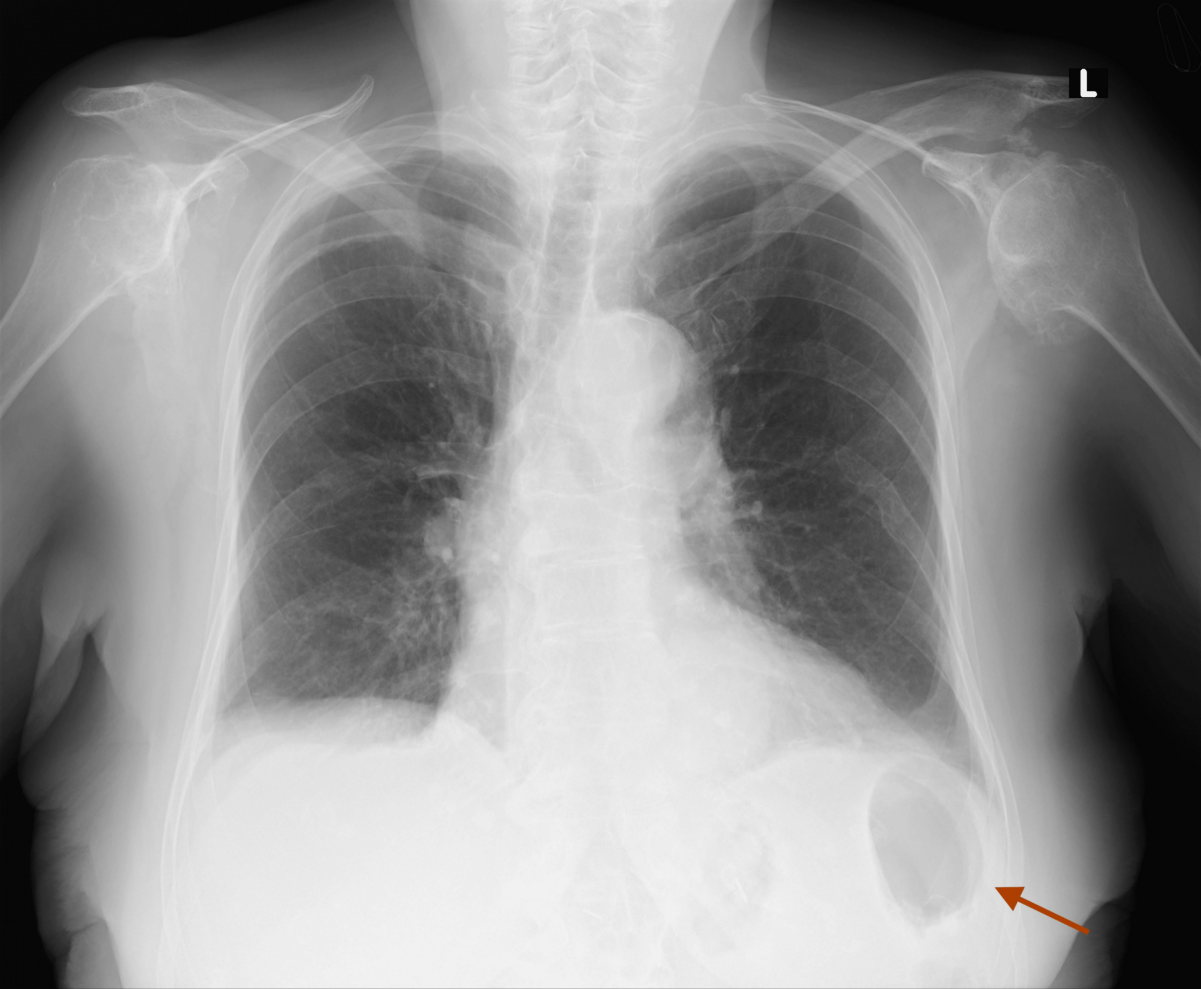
Follow-up chest X-ray with correct stomach position (gastric bubble).
